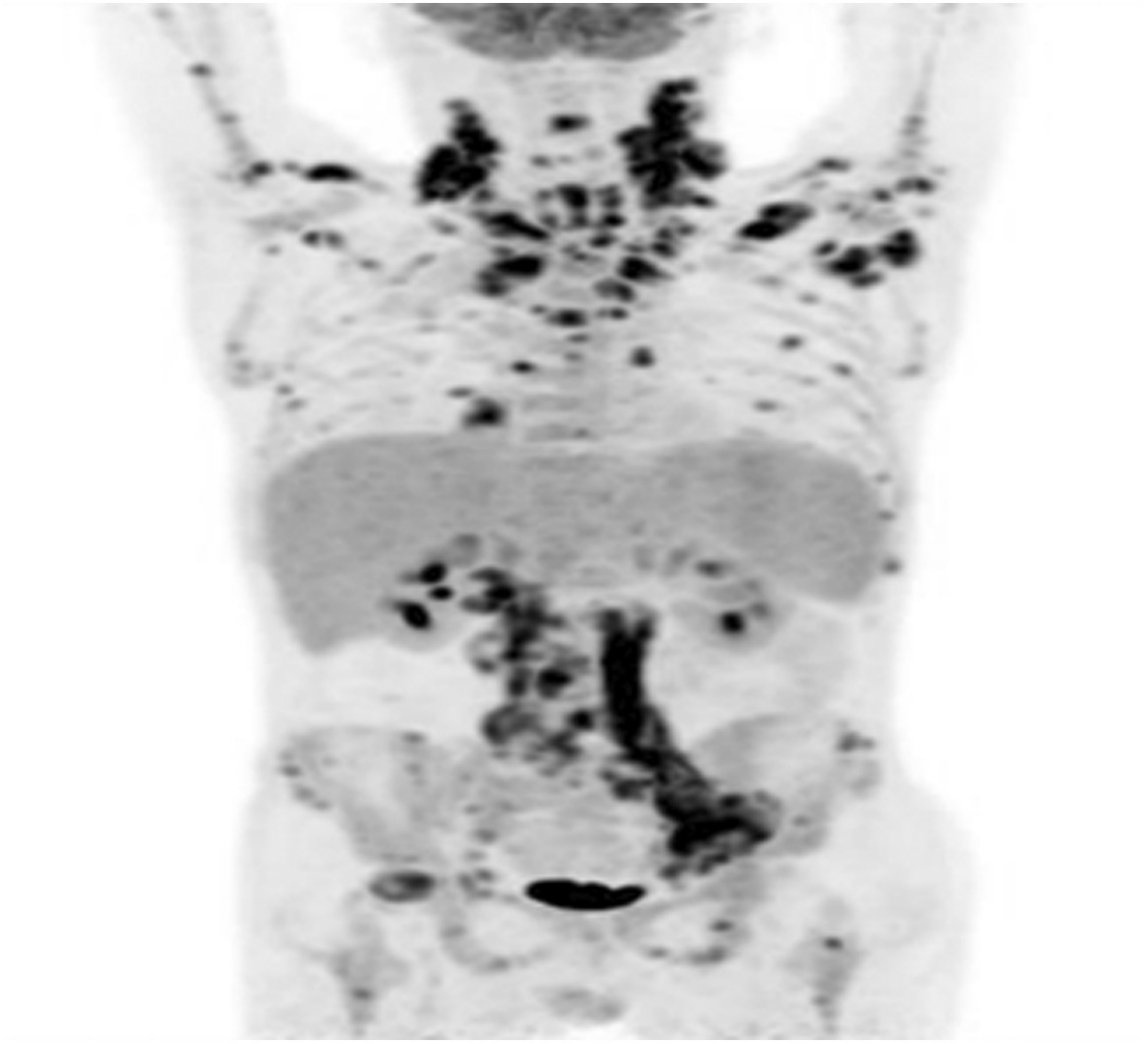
PET/CT image shows increased FDG uptake in multiple parts of the skeleton, multiple lymph nodes, and multiple irregular mass of upper pulmonary lobe.
